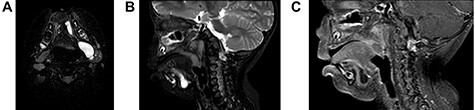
(A) On axial view, MRI demonstrated multi-lobulated cystic mass, which showed hypersignal intensity on T2 weighted image involving left sublingual, submandibular, pharyngeal and parapharyngeal spaces; (B) On sagittal view, MRI demonstrated multi-lobulated cystic mass, which showed hypersignal intensity on T2 weighted image involving left sublingual and submandibular spaces; (C) On sagittal view, MRI demonstrated multi-lobulated cystic mass, which showed hyposignal intensity on T1 fat suppression with gadolinium involving left sublingual and submandibular spaces.
